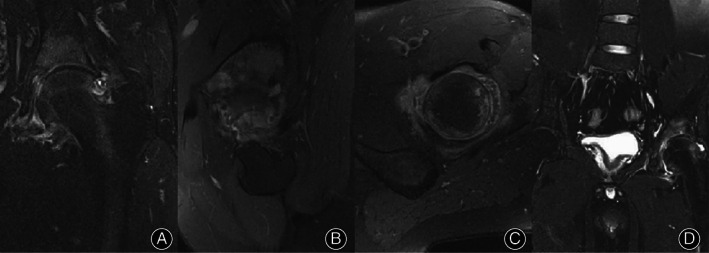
The MRI of hip PVNS received arthroscopy. (A, B, C) Preoperative MRI of the left hip. (D) MRI after operation
